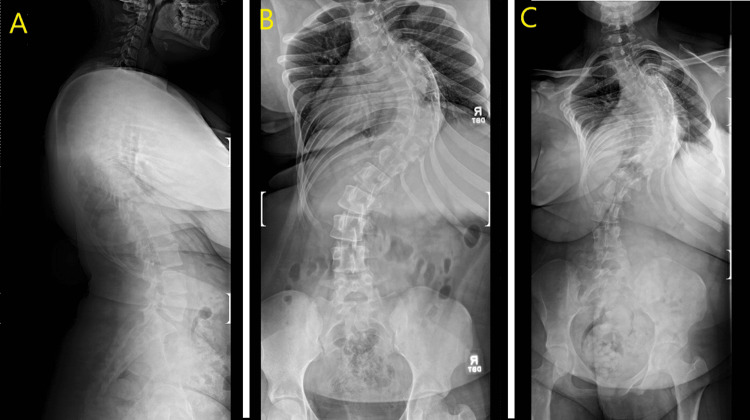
Pre-operative standing X-ray showing A) sagittal view, B) anteroposterior (AP) view, and C) posteroanterior (PA) view of spinal deformity
